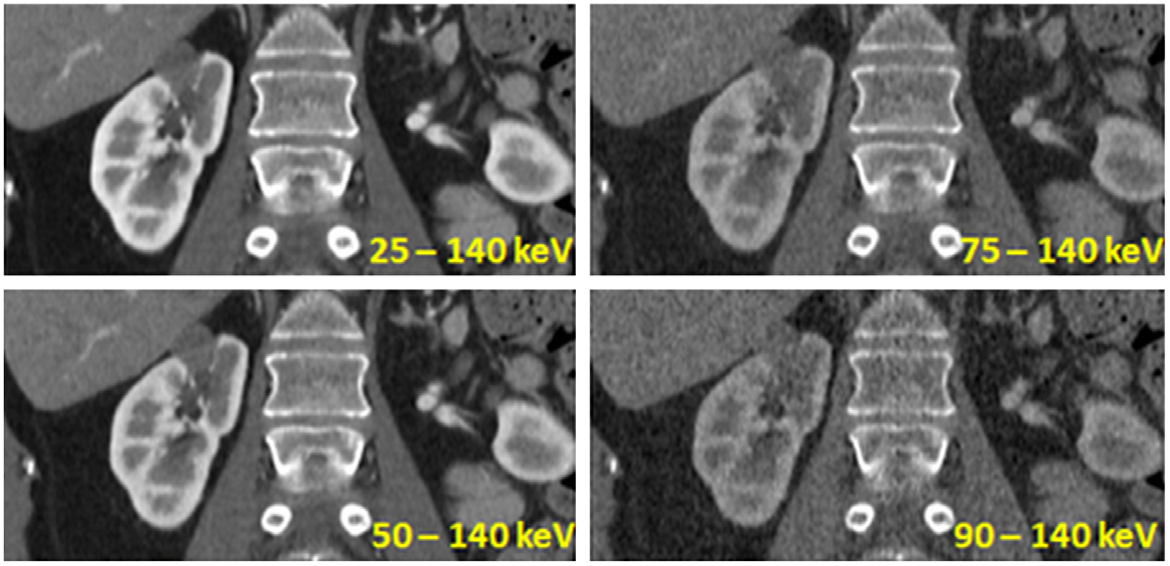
Contrast-enhanced kidney scan acquired with a preclinical photon counting CT prototype with four low-energy thresholds (25, 50, 75, and 90 keV) as indicated in Fig. 25, operated at an x-ray tube voltage of 140 kVp. The higher the lower energy threshold is, the lower is the iodine contrast, and the higher is the image noise in the reconstructed images, because fewer low-energy x-ray photons contribute to the image. Images courtesy of National Institutes of Health (NIH), Bethesda, MD, USA.
